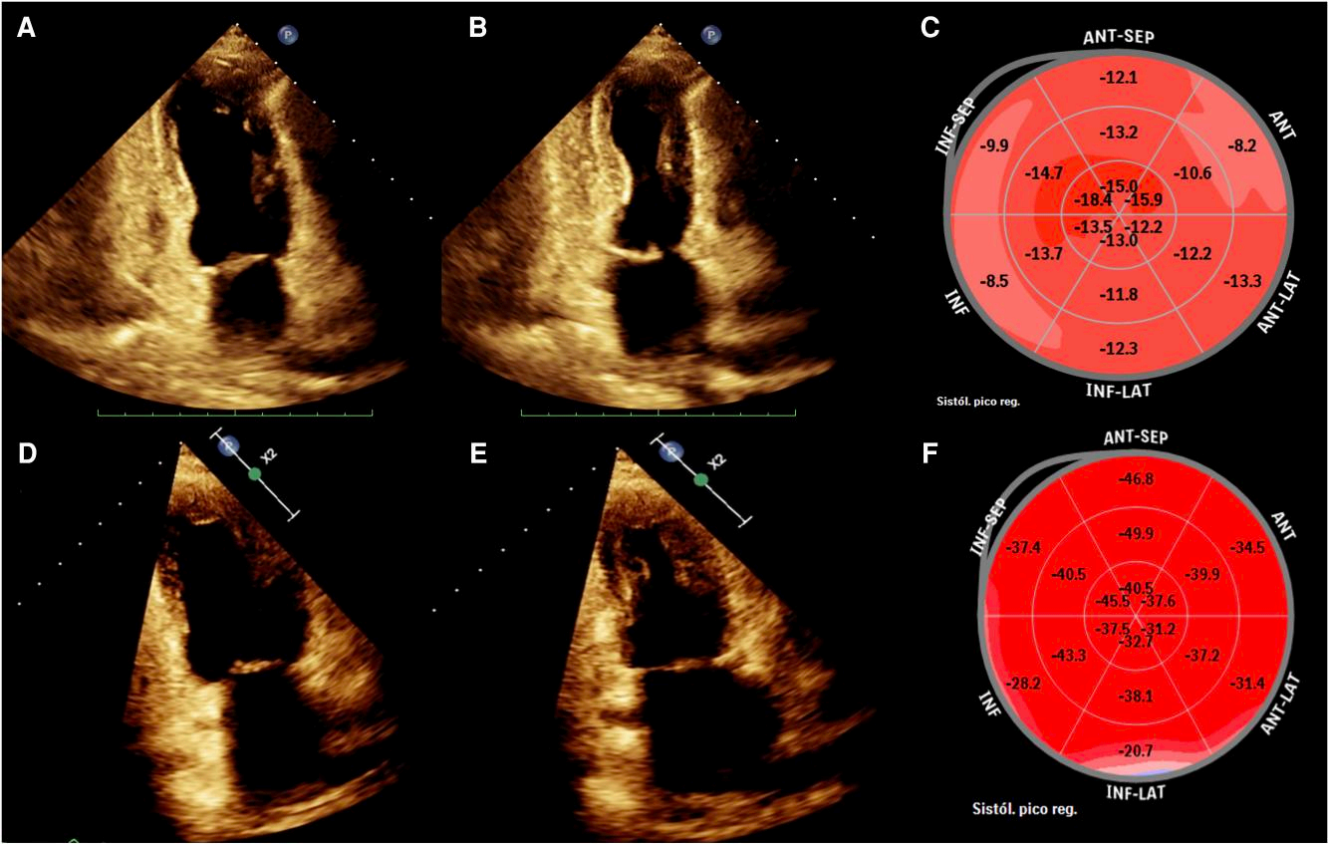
Transthoracic echocardiogram during the acute event (*A* and *B*) showing basal hyperkinesia and apical akinesia, and at the three-month follow-up (*D–F*) demonstrating normalization of regional wall motion. Note that the GLS was reduced in the basal segments, which were otherwise normal, compared to the apical segment where there was akinesia during the acute event (*C*). This may be secondary to disproportionate exposure or an exaggerated response to catecholamines in the basal segments, leading to temporary dysfunction or reduced contractility, as reflected by the reduced strain in these segments.
